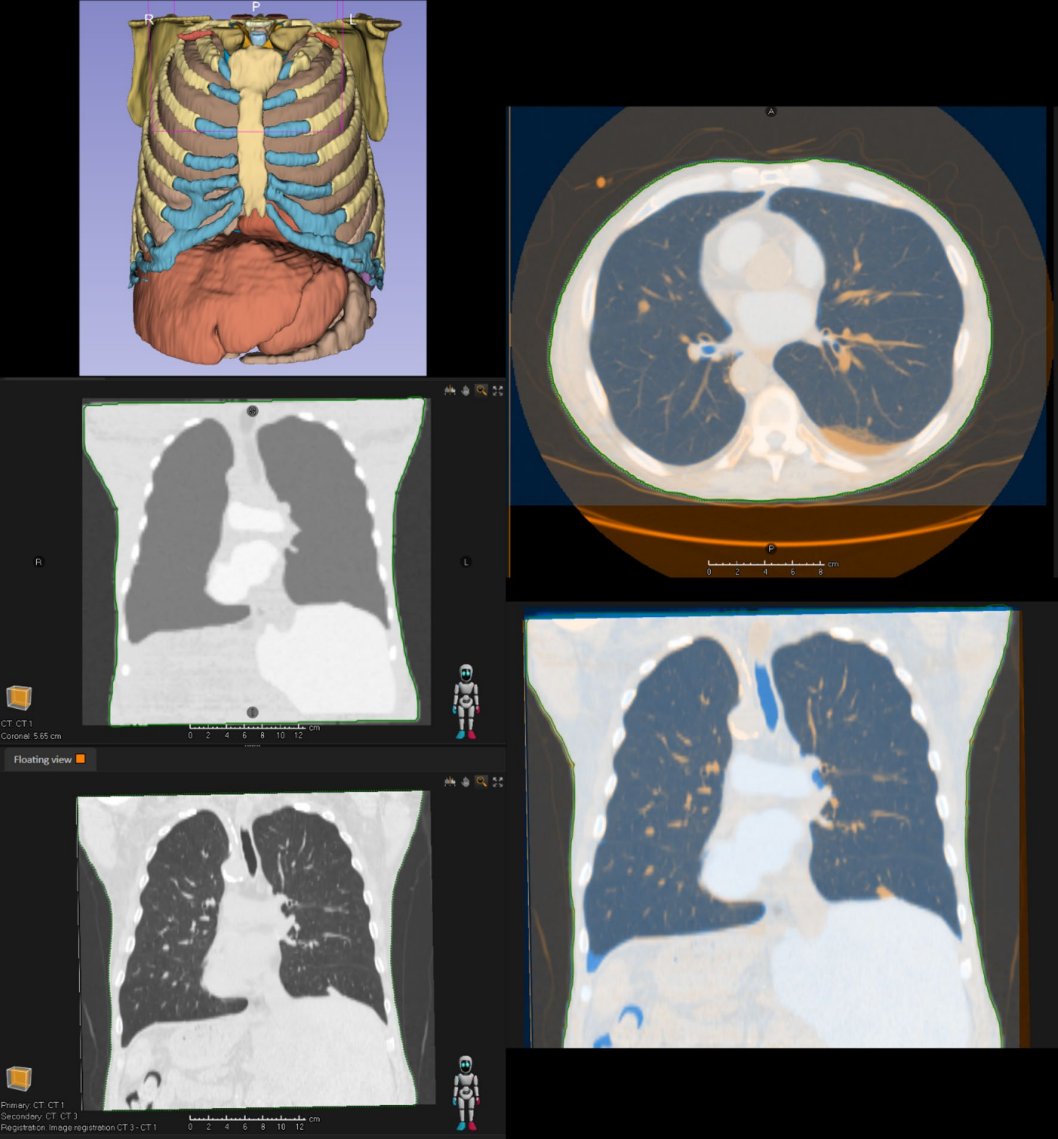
Comparison of the original patient CT and the synthetic dataset generated using DICOMator. Top left—The 3D anatomical model used to generate the synthetic dataset. Middle Left—The synthetic CT dataset. Bottom Left—The original “ground-truth” patient CT dataset. Right—The image fusion between the two datasets as shown in Raystation
